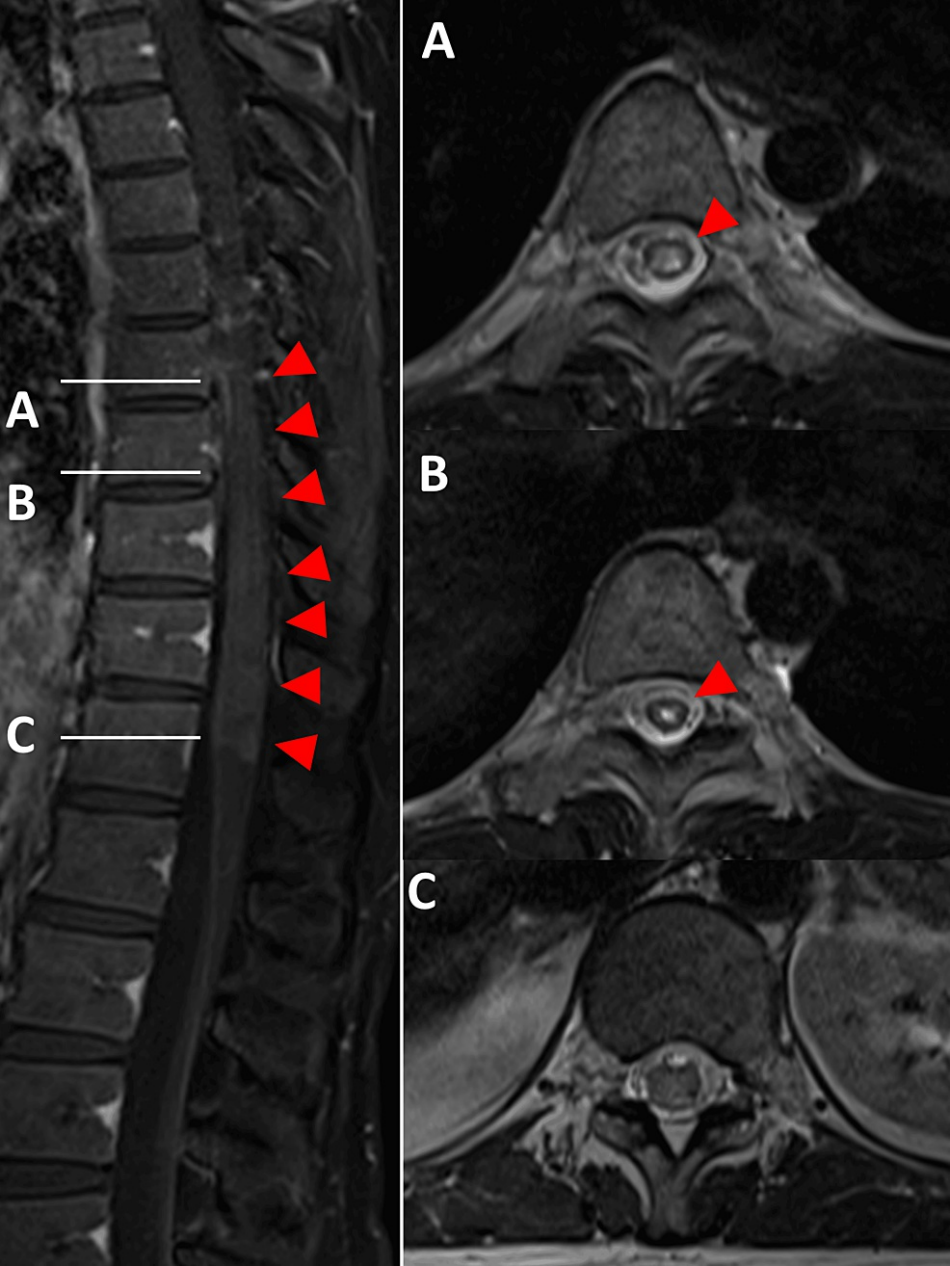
Thoracic MRI Gd-T1WI in the sagittal plane and T2WI in the axial plane at third attack Thoracic MRI revealed longitudinal lesions with gadolinium enhancement (red arrows), extending from the thoracic vertebral bodies (T) 8 to T12 levels. The respective heights indicated in the sagittal section of T2WI correspond to axial slices at the following levels: (A) is at T8, (B) is at T9, and (C) is at T12. Gd-T1WI: Gadolinium-enhanced T1-weighted; T2WI: T2-weighted imaging
